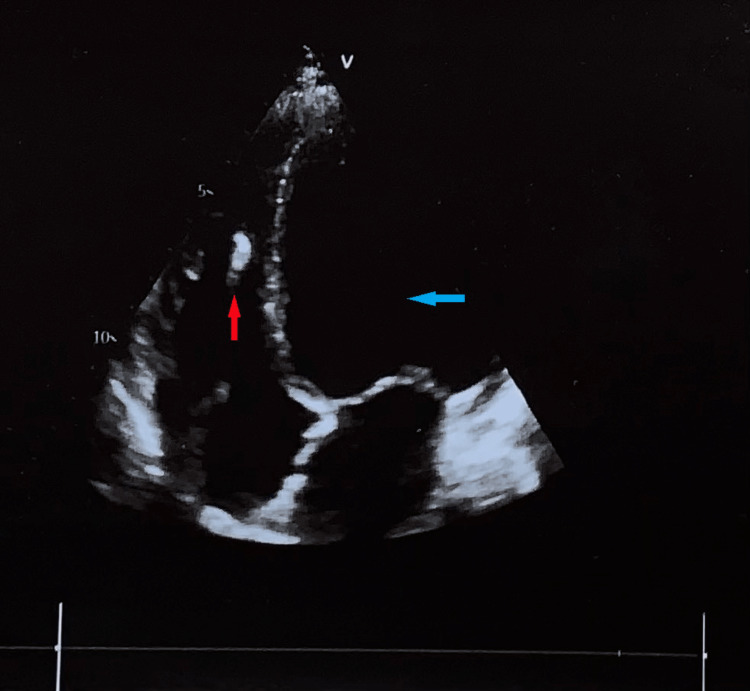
Transthoracic 2D ECHO, apical 4-chamber view showing dilated left ventricle (blue arrow). The ICD lead is visible (red arrow).
